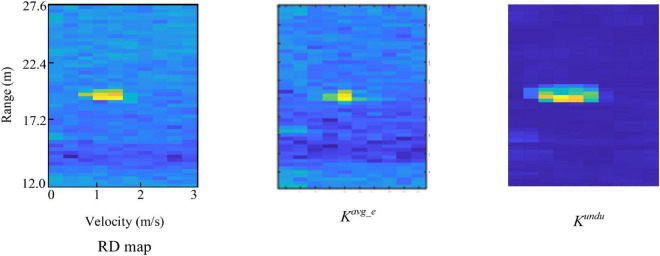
K^avg_e^* and *K^undu^* extracted from the same region-of-interest (RoI) in an range-Doppler (RD) map.
